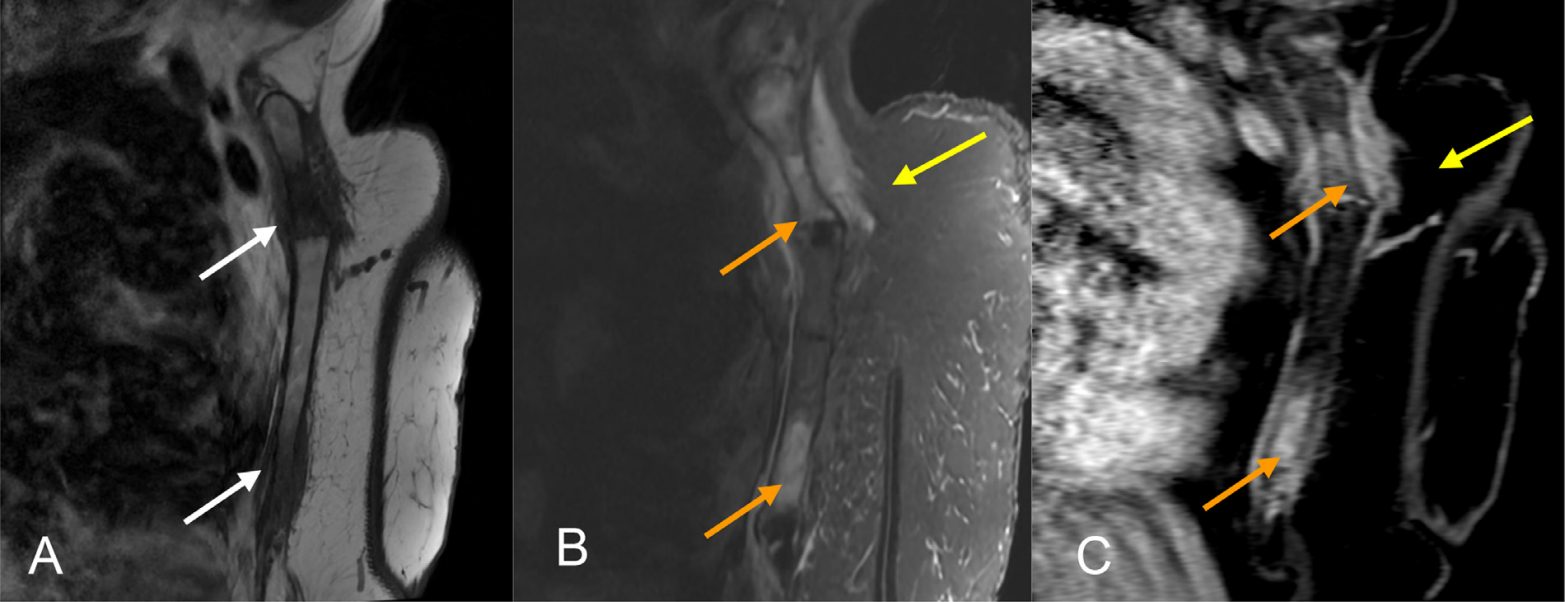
MRI chest sagittal: (A) T1, (B) STIR, (C) LAVA (T1 FS post contrast). (A) Abnormal T1 hypointense marrow replacement at the manubriosternal junction and lower sternum (white arrows) (B&C) with enhancing edema (orange arrows) and adjacent soft tissue inflammation (yellow arrows). No fluid collection or sinus tracts demonstrated.
